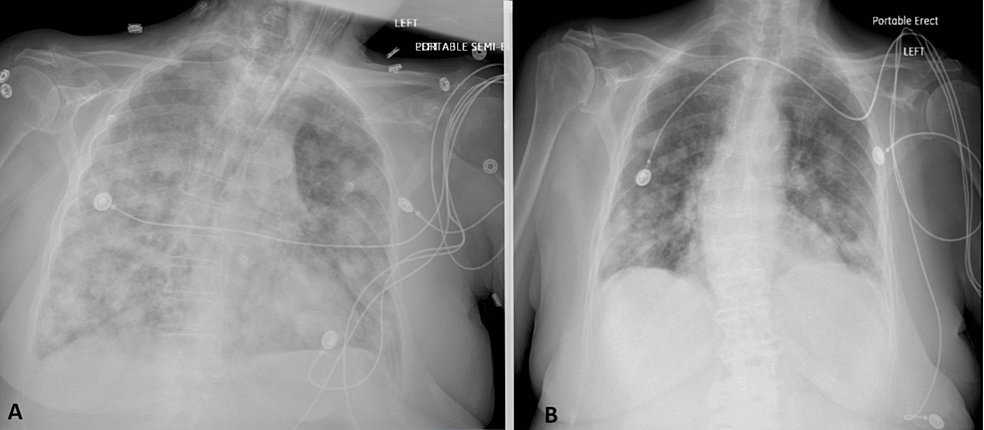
CXR images Image A shows intubated with new pulmonary infiltrates during the second admission on top of lung nodules. B CXR on the first day of previous admission with pulmonary nodules without infiltrates. CXR: Chest X-ray
